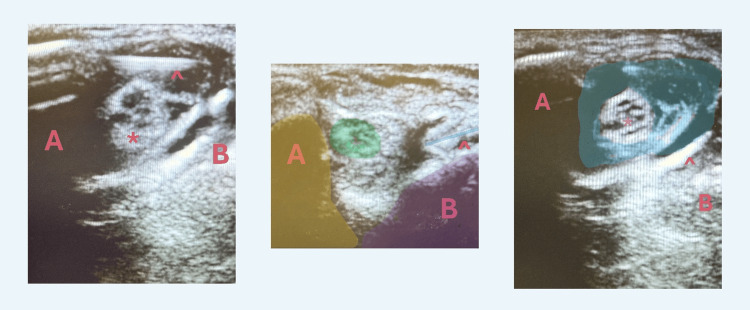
Ultrasound image demonstrating hydrodissection around ulnar nerve (A) medial epicondyle, (B) olecranon, (*) ulnar nerve, (^) needle, blue highlight =  injectate demonstrating hydrodissection around nerve
